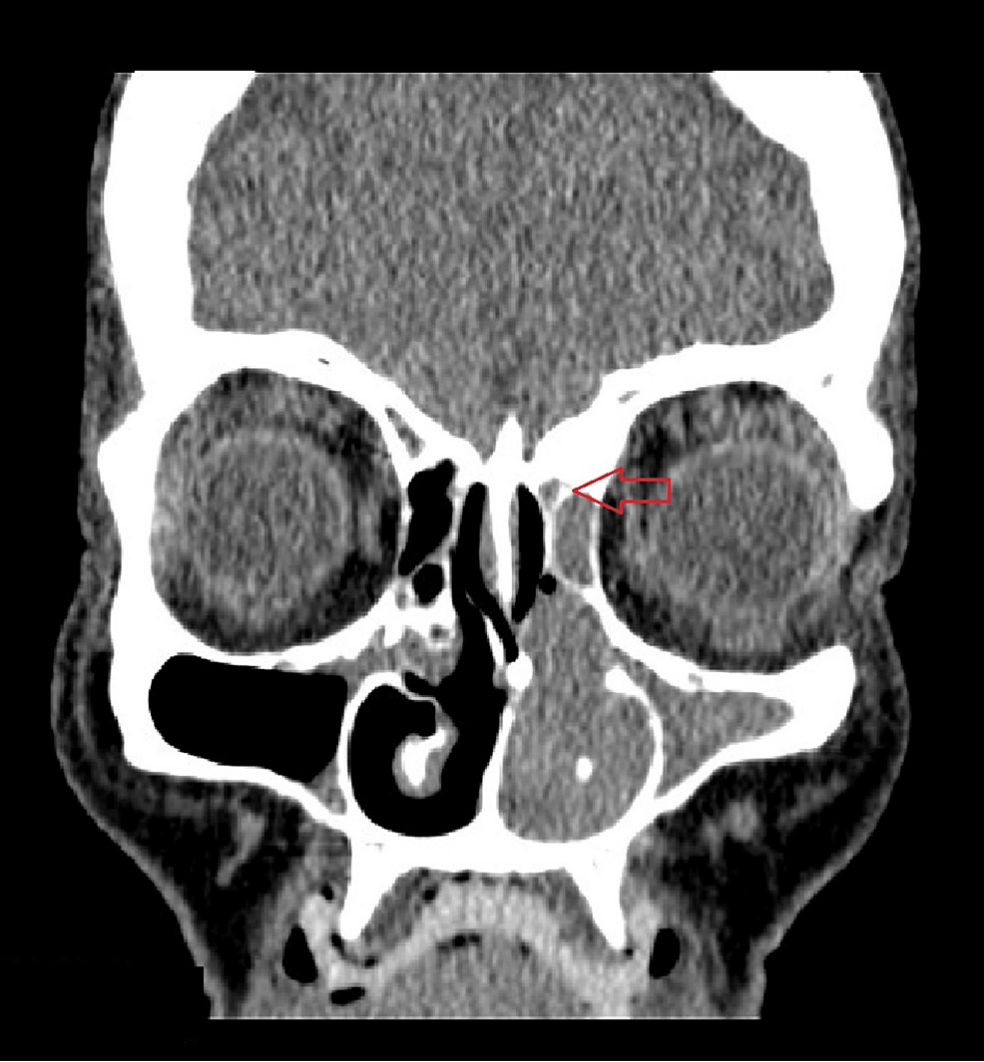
Coronal CT scan showing a mass (red arrow) extending superiorly to the ethmoid sinus
